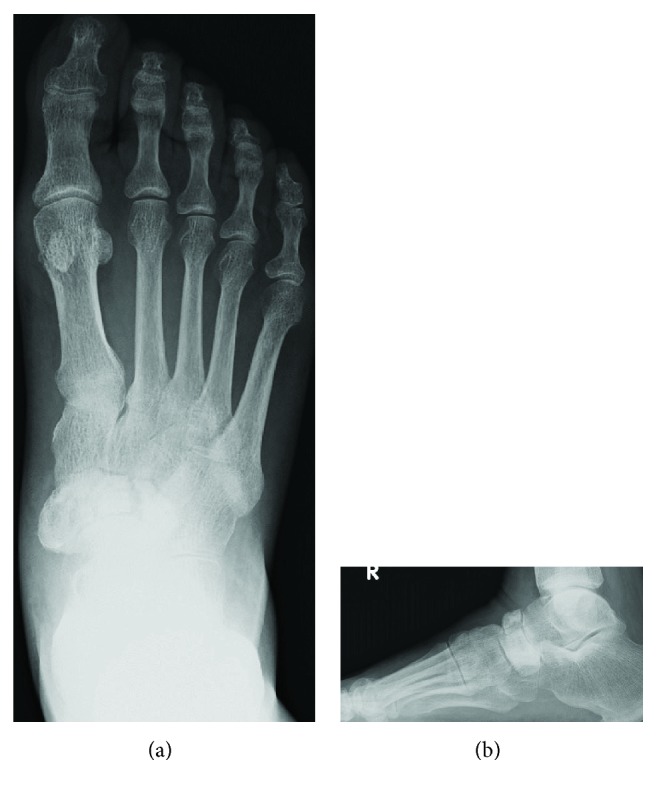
Frontal and lateral radiographs show increased radiodensity, dorsal protrusion, and fragmentation of the tarsal navicular.
